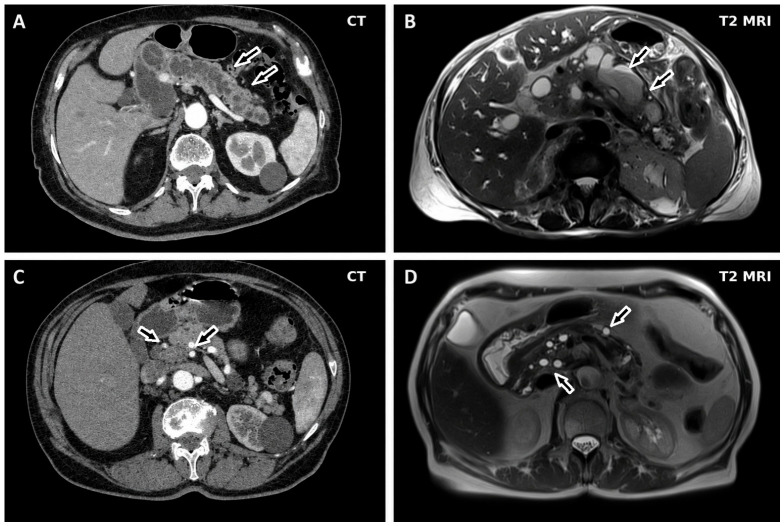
Representative cross-sectional imaging findings of main-duct and branch-duct intraductal papillary mucinous neoplasms (IPMNs). (A,B) Contrast-enhanced computed tomography (CT) (A) and axial T2-weighted magnetic resonance imaging (MRI) (B) from the same patient with main-duct IPMN (MD-IPMN), demonstrating diffuse dilatation of the main pancreatic duct (indicated by white arrows). (C,D) Contrast-enhanced CT (C) and axial T2-weighted MRI (D) from a patient with branch-duct IPMN (BD-IPMN), showing multiple fluid-signal cystic lesions arising from the branch ducts and communicating with the main pancreatic duct (indicated by white arrows).
